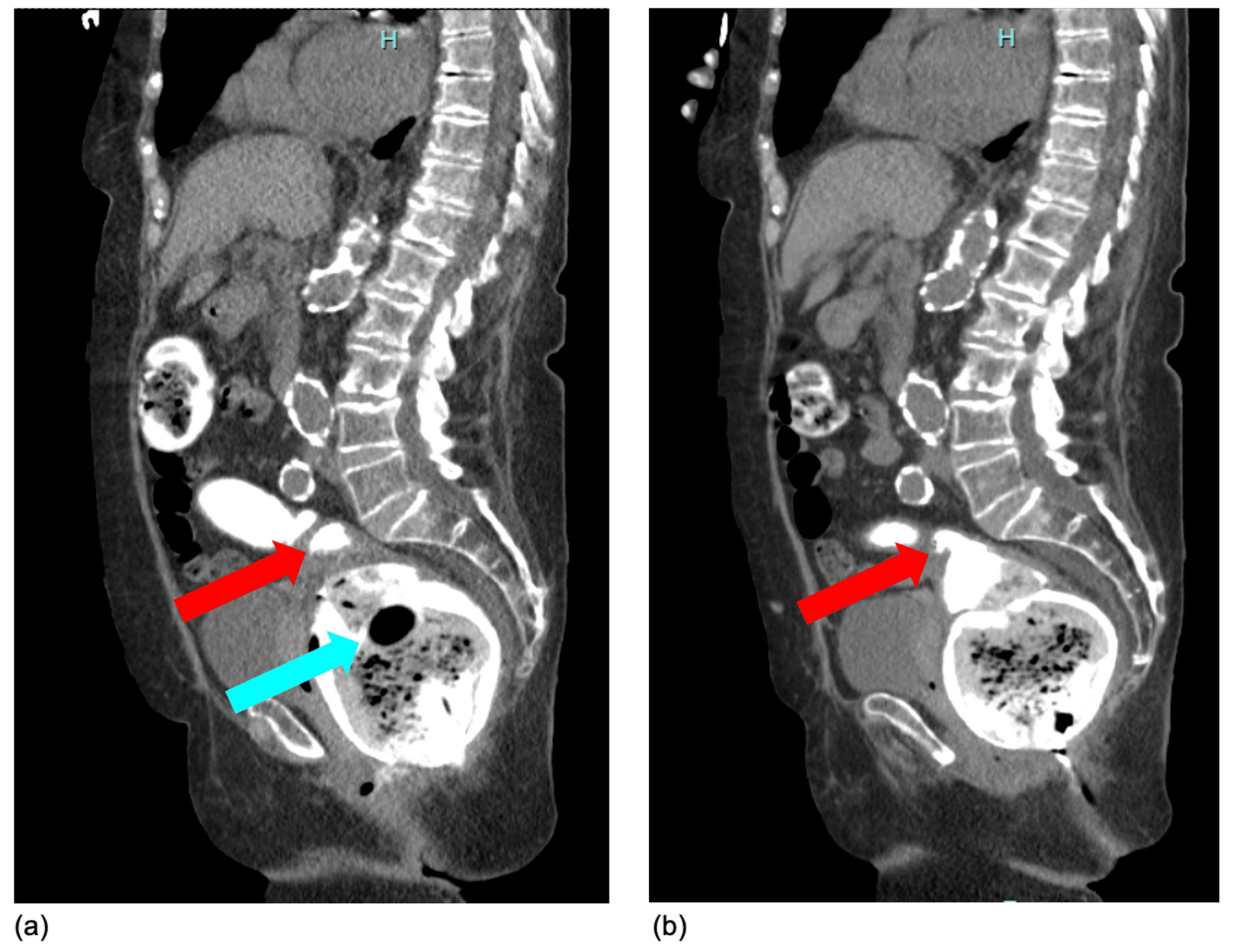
Sagittal views of computed tomography of the abdomen/pelvis for Case 1 Sagittal views of computed tomography (CT) of the abdomen/pelvis with rectal contrast on hospital day 65, after up to an estimated 19 days of FMS use, before (a) and after (b) evacuation of the rectum, showing focal anterior extension of enteric contrast at the level of the rectum towards the vagina. Red arrow = area of focal contrast; blue arrow = rectal tube
